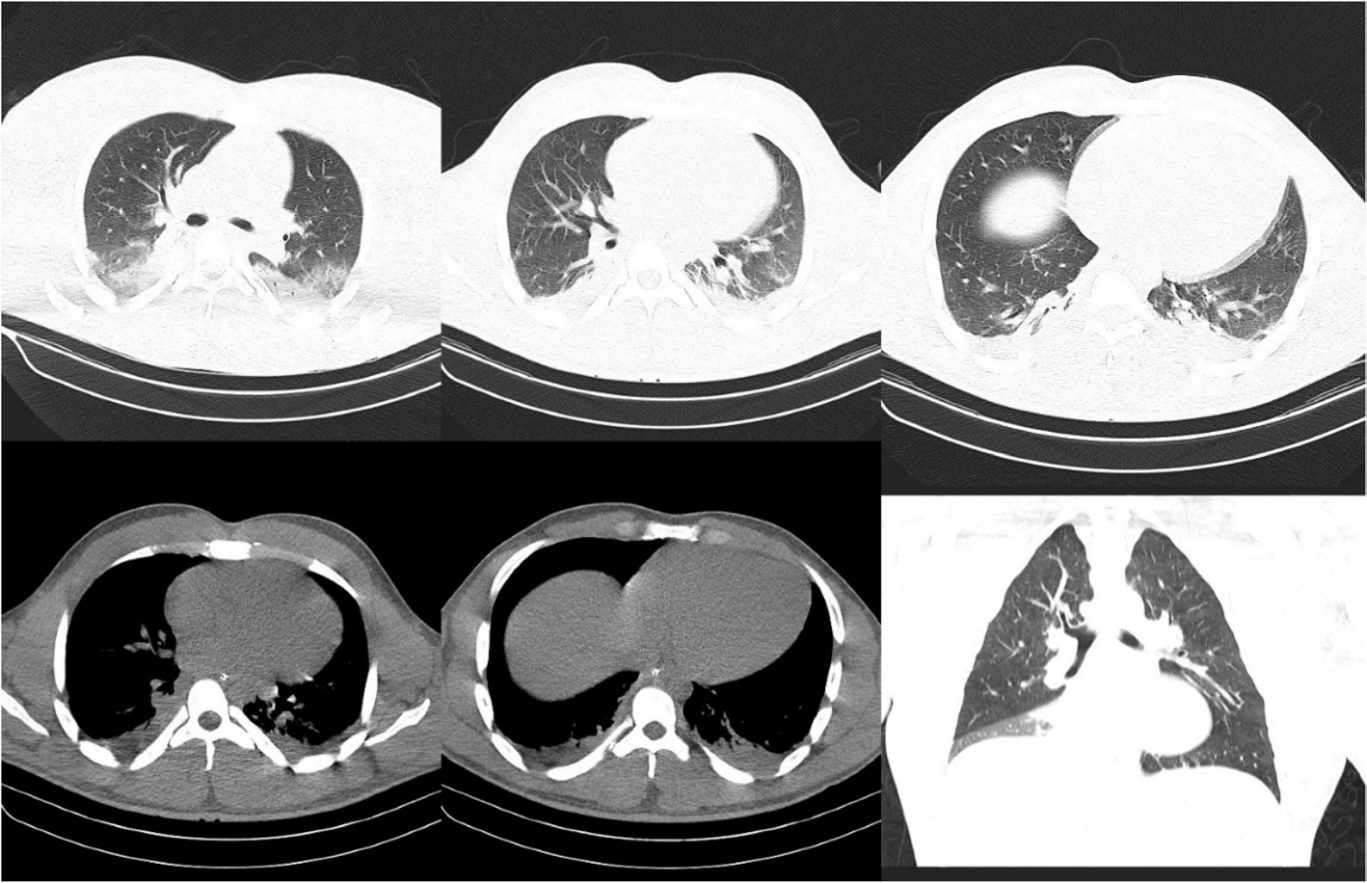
Chest CT scan; consolidations in the dependent areas of lungs are indicative of aspiration pneumonia
